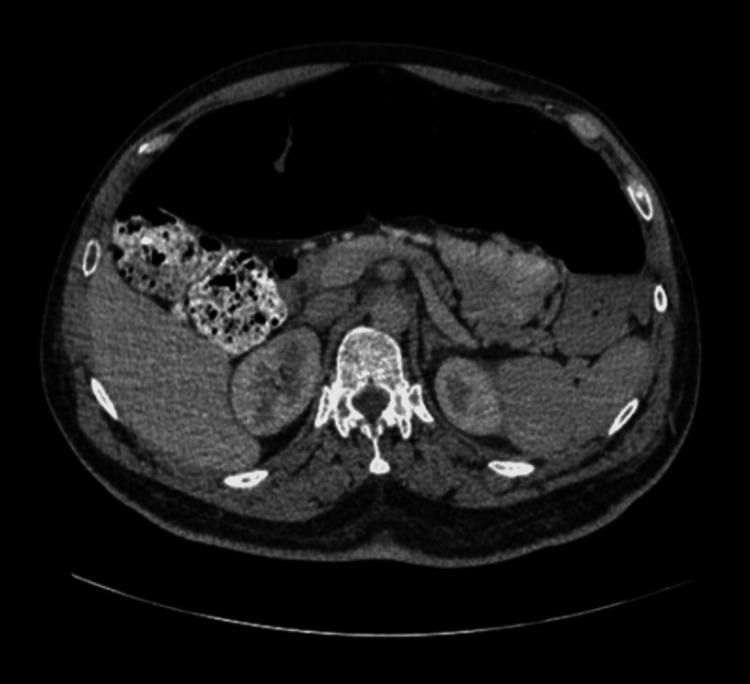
CT of the abdomen with contrast showing bowel dysmotility and distension
